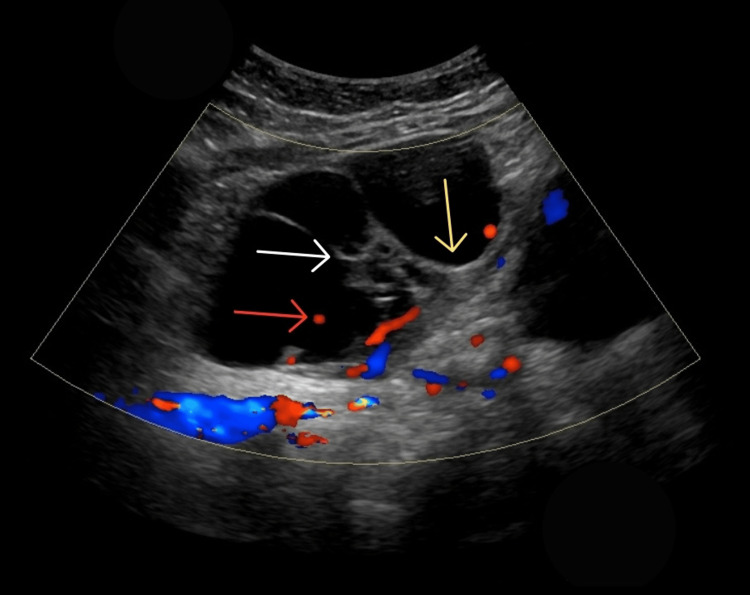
Pelvic ultrasound. Pelvic ultrasound demonstrating a multiloculated left ovarian cystic lesion with an internal solid mural nodule (white arrow) and thick septations (yellow arrow). Color Doppler reveals internal vascularity within the lesion (red arrow), raising suspicion for a neoplastic ovarian mass.
